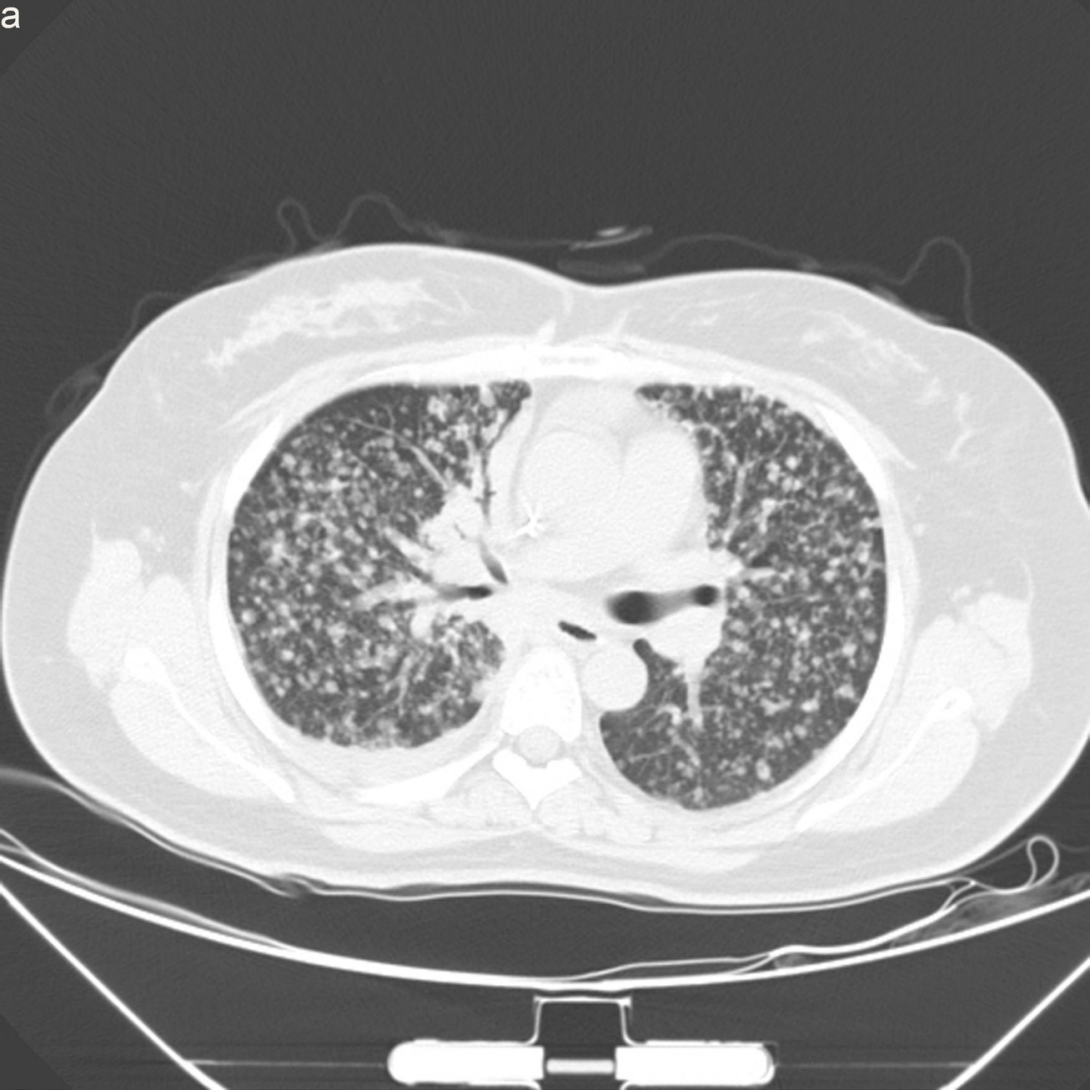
Computed tomography scan images of lung prior and post-AZD9291 treatment a. Disease progressed in Dec,2015 after treatment of erlotinib and chemotherapy, prior-AZD9291. Patient had symptoms of cough and short of breath. b. Partial response after one month of AZD9291 treatment. Symptoms were much relieved.
